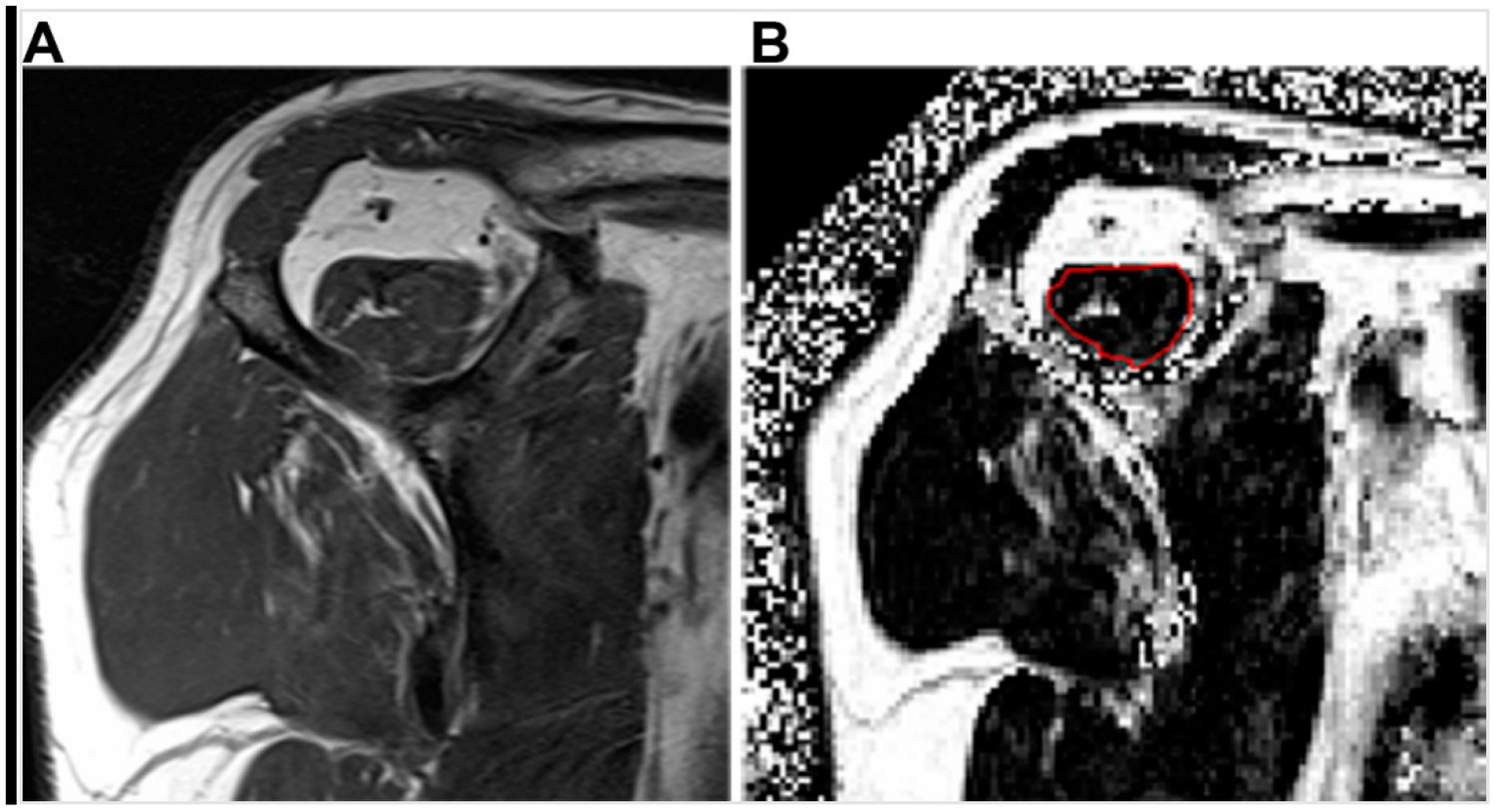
Examples of rotator cuff muscle fatty infiltration classification on magnetic resonance imaging (MRI). (A) Oblique sagittal T1-weighted MRI shows a supraspinatus muscle with streaks of internal fat, consistent with semi-quantitative Goutallier grade 1 fatty infiltration. (B) Quantitative oblique sagittal 6-point Dixon fat fraction map MRI following manual image segmentation calculates an amount of 10.5% supraspinatus muscle fatty infiltration within the region of interest (red circle).
